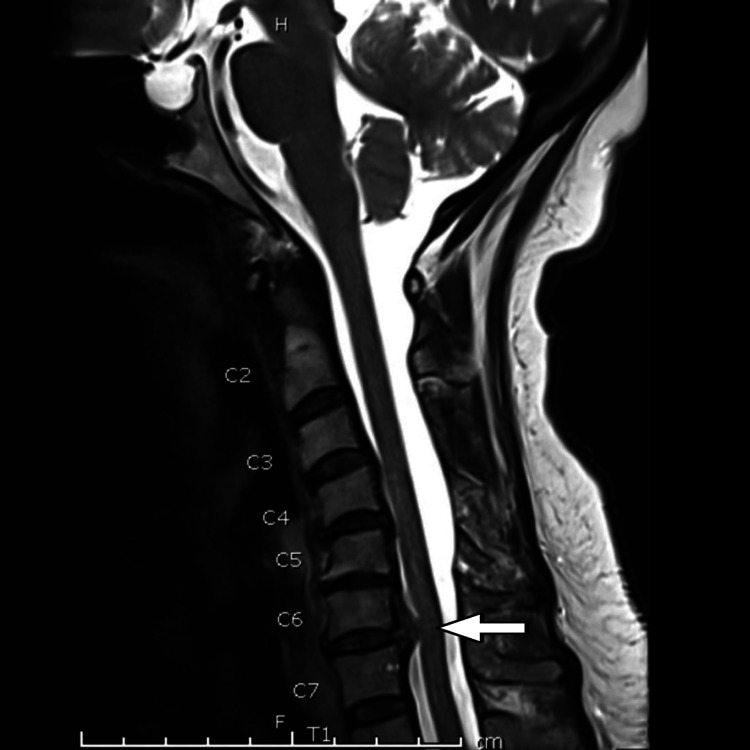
T2-weighted MRI of the cervical spine showing degenerative spondyloarthropathy at C6/C7 with spinal canal compression and cord indentation The arrow indicates a degenerative disc-osteophyte complex at the C6/C7 level causing spinal canal narrowing and indentation of the cord. Cord signal changes were absent. MRI: magnetic resonance imaging
